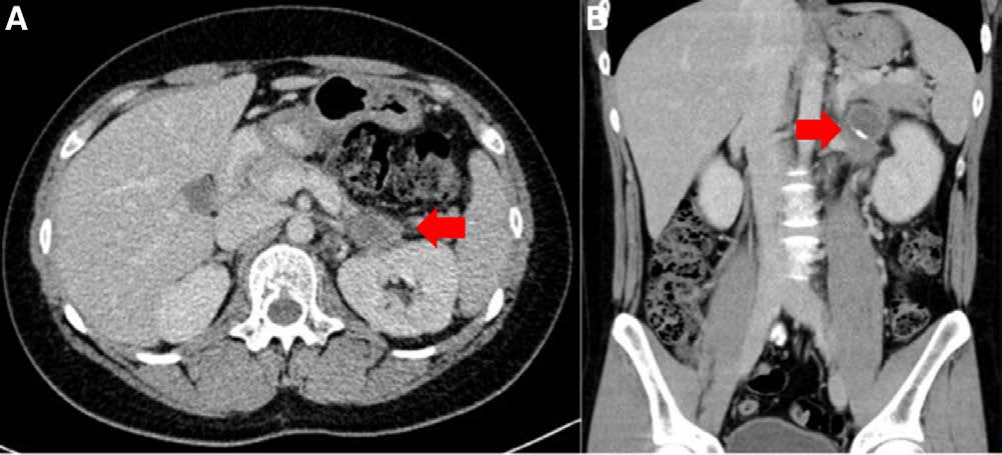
(A, B) Abdominal computed tomography. After 6 months of chemotherapy, the tumor size decreased while encasing the left renal vessels (arrow).
